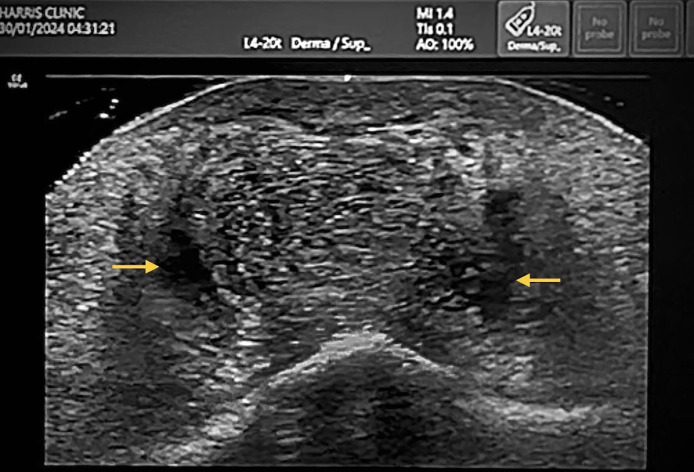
Ultrasound of the chin demonstrating chronic filler-related changes, including persistent hyaluronic acid material (arrows), interstitial edema, and hyperechoic fibrotic septa.
